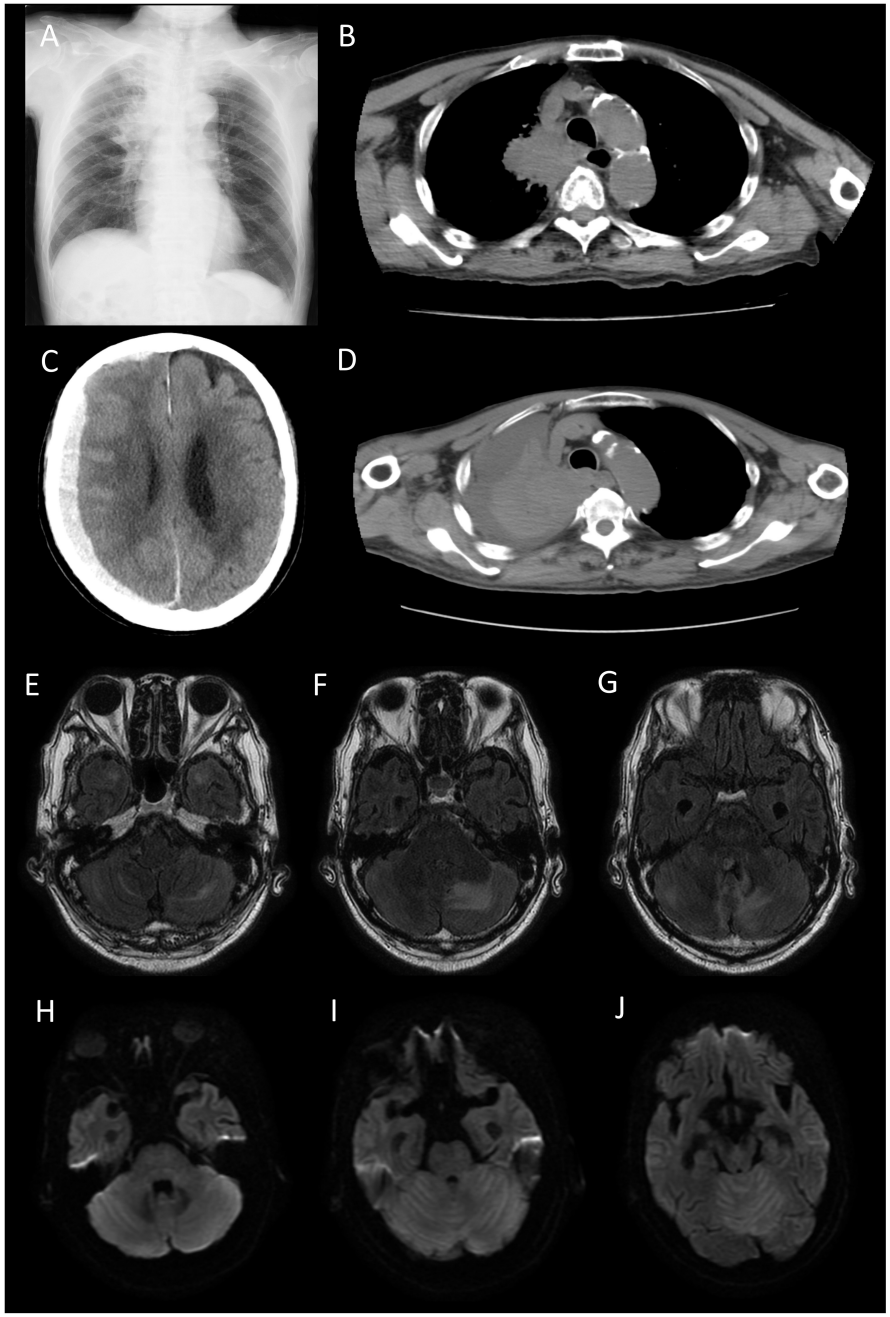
Chest radiograph (A) and computed tomography (CT) scan (B) on admission. A right hilar tumorous lesion was observed. Brain CT (C) and chest CT (D) on 10 days after admission. Cryptogenic acute subdural hematoma and increased pleural effusion were apparent. Fluid-attenuated inversion recovery (FLAIR) (E–G) and Diffusion-weighted image (DWI) (H–J) on admission. FLAIR showed T2 hyperintensities in the bilateral temporal polar white matter and light-predominant edematous cerebellar lesions. DWI showed high intensities in the whole cerebellum but no apparent abnormalities in the bilateral temporal poles. No tumorous lesions were apparent.
